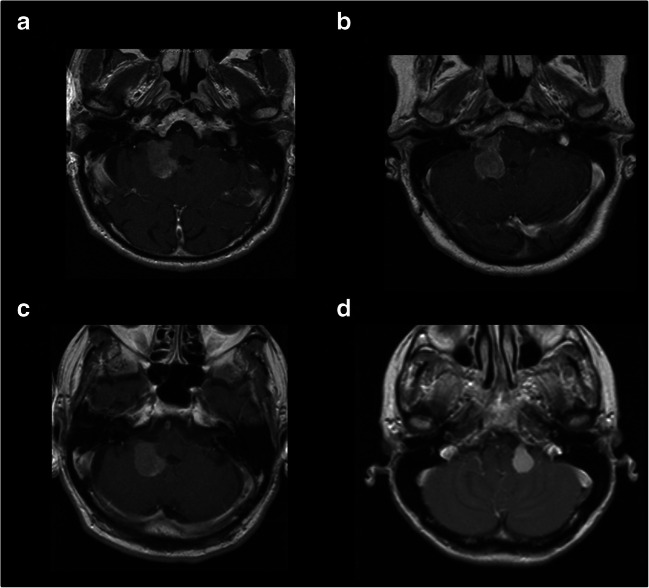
Axial MRI (A, B, C, D) of different Type 2 choroid plexus papillomas: tumor portions are present in the cerebellomedullary angle (inferior to the line between the pons and medullar oblongata), without any tumor portions in the cerebellopontine angle
